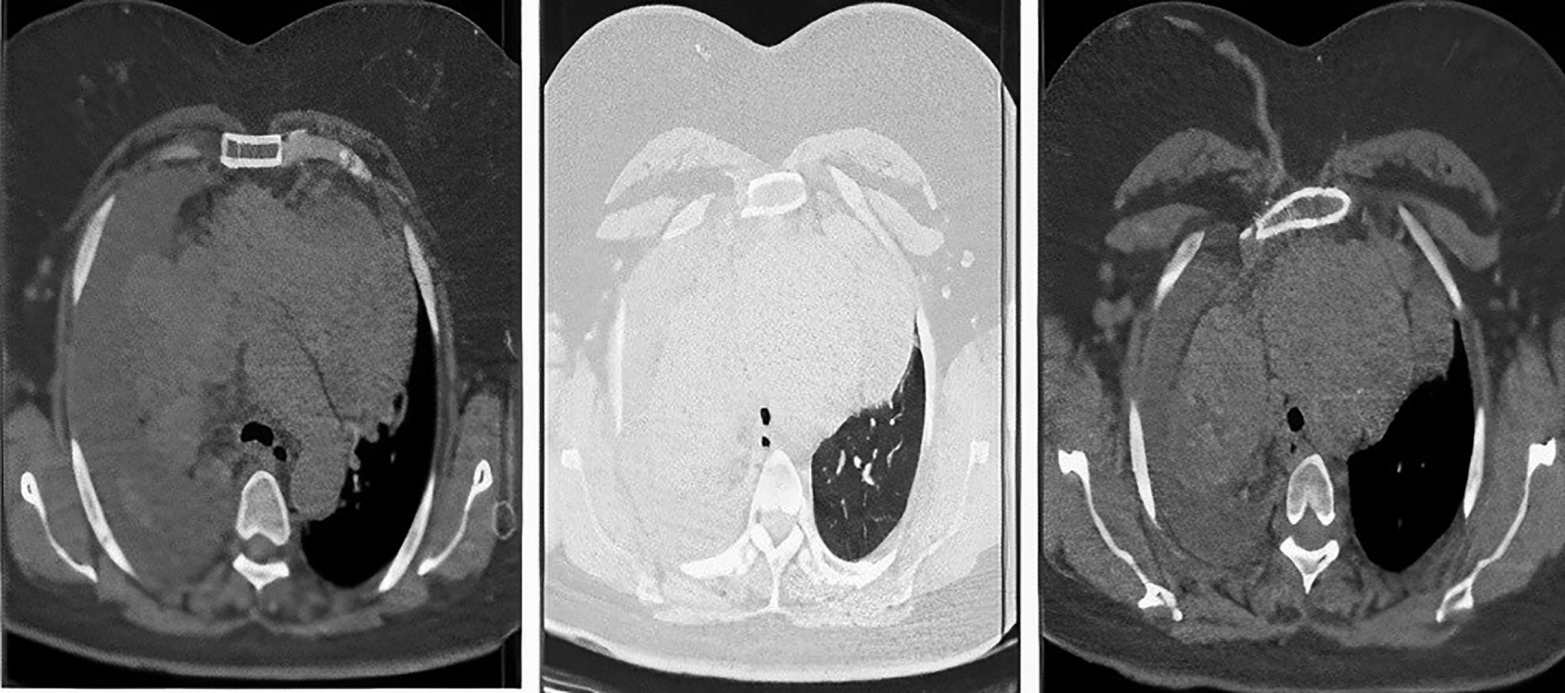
Lung high‐resolution computed tomography (HRCT), pleural fluid.
